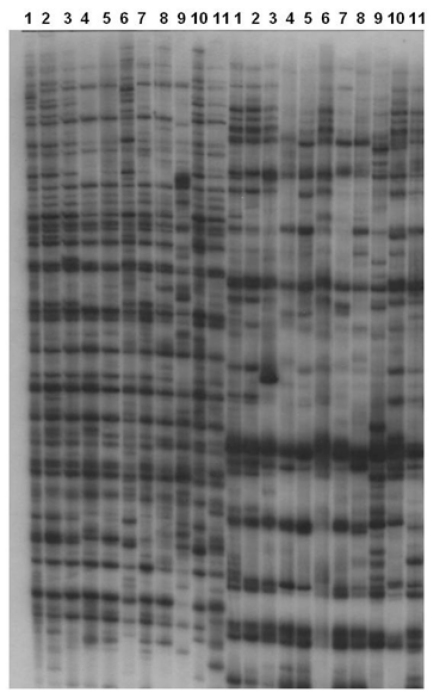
AFLP Autoradiogram of pigeon pea cyst nematode *Heterodera cajani* with *EcoRI* (+AAG) + *MseI*, (+CAG) and *EcoRI* (+AAA) + *MseI* (CTA). Lane 1 to 11:
